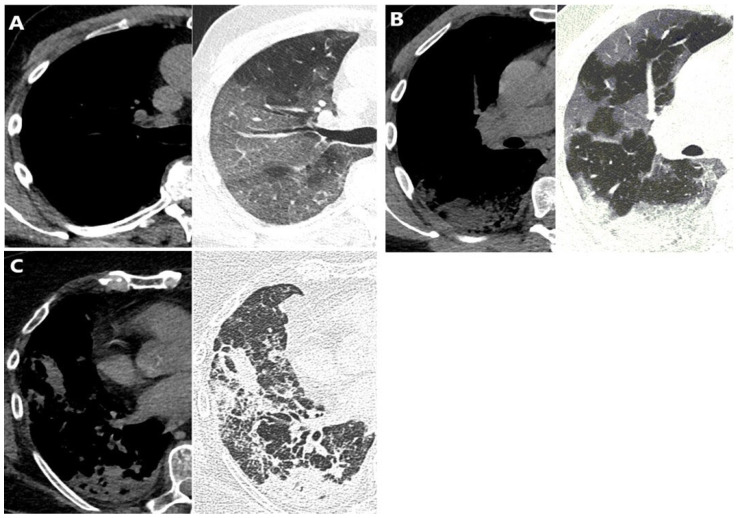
Predominant lung pattern on mediastinal (left) and lung (right) reconstructions of axial CT scans at the level of right lover lobe in three different patients: (A) ground-glass opacity, (B) mixed pattern (combination of ground-glass opacity and consolidation), (C) consolidation.
